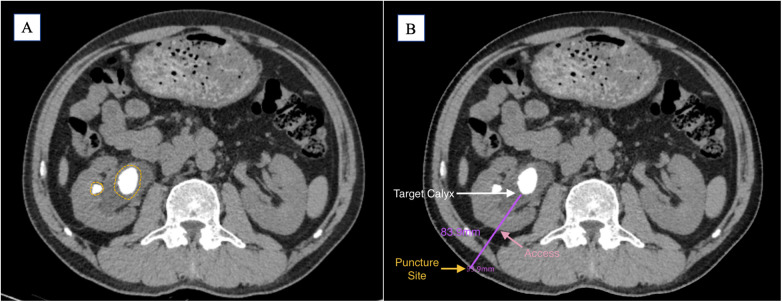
(A) Preoperative CT imaging shows the main stone located in the renal pelvis, with a small stone visible in the mid-anterior calyx (yellow circle). (B) Based on the preoperative findings, the target calyx is selected (white arrow); the shortest perpendicular path to the skin is indicated (pink arrow); the puncture point is marked (yellow arrow); the estimated puncture tract length is 83.9 mm (purple line).
